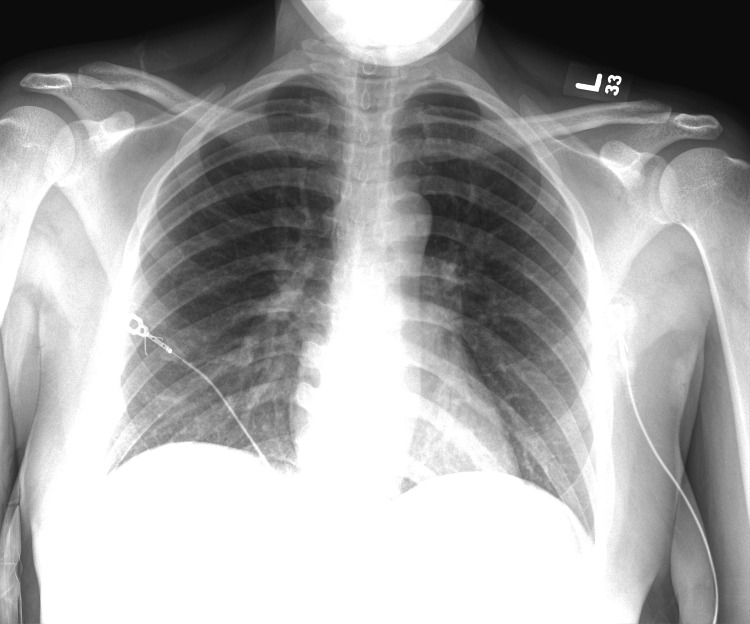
Normal chest radiograph at the initial presentation
